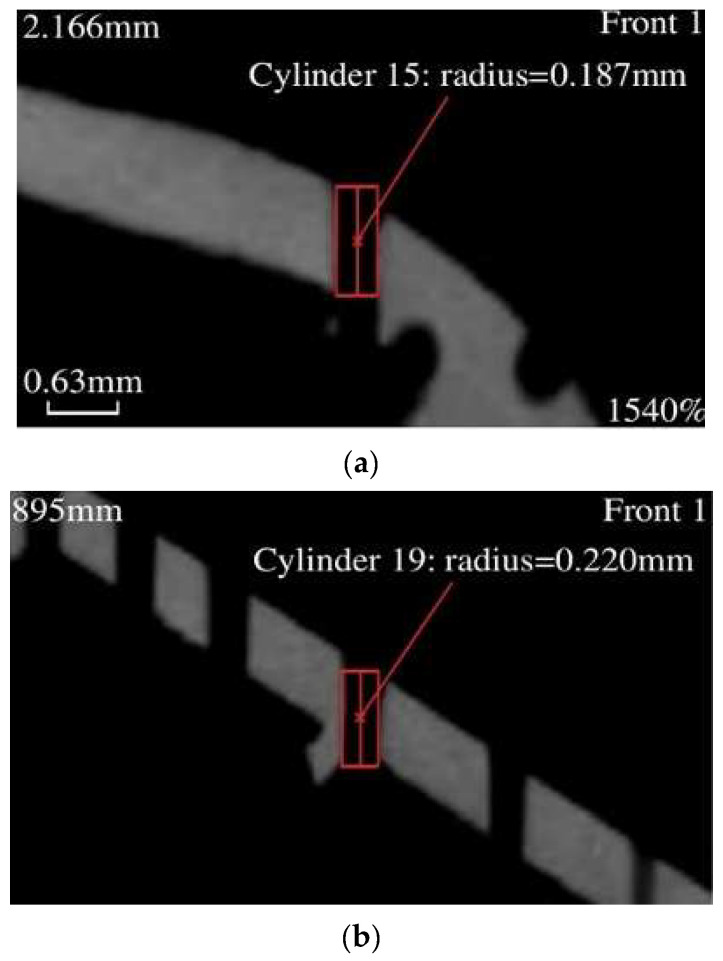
Blade profile image from industrial CT [66]. (a) Measurement results of squealer tip from industrial computer tomography (CT). (b) Measurement results of 2nd row cooling hole from industrial computer tomography (CT).
